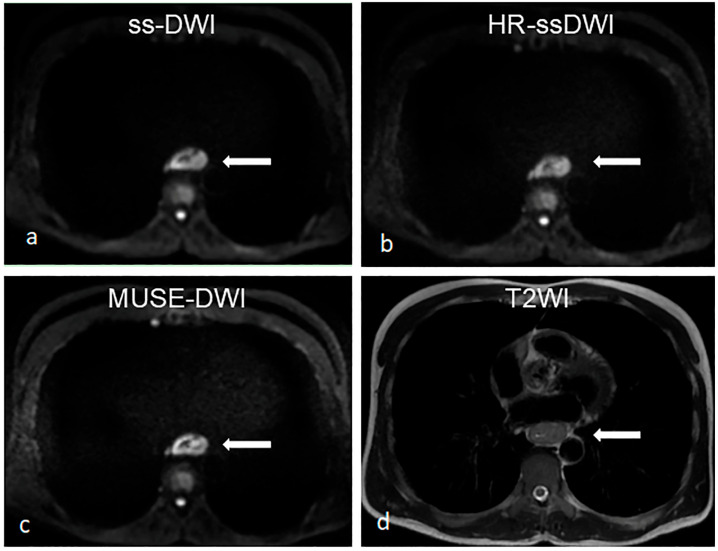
Images acquired from a 48-year-old male esophageal cancer patient with ss-DWI (a), HR-ssDWI (b), MUSE-DWI (c), and T2-weighted (d) sequences; the image quality, esophageal contour, lesion conspicuity and image distortion of MUSE-DWI are all superior to HR-ssDWI. Compared with ss-DWI, MUSE-DWI provides a better visualization of fine structures. The arrow indicates the esophageal tumor.
